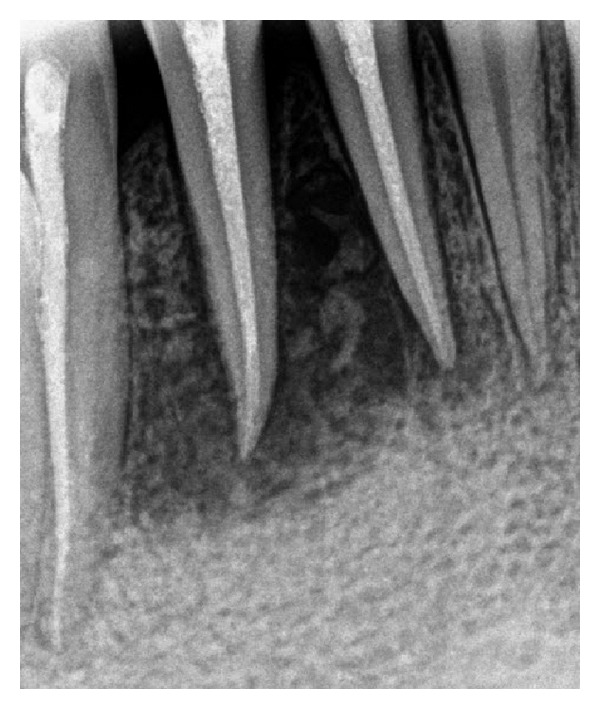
6 months postoperative radiograph.
